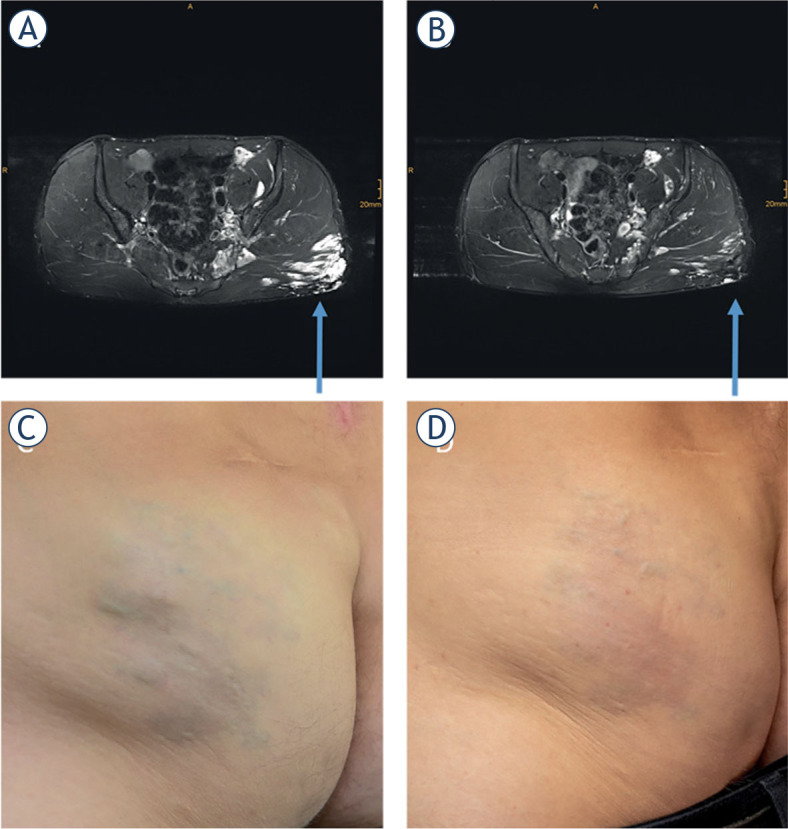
Patient treated by BEST. Axial T2-weighted, fat-saturated MRI with hyperintense (arrow) gluteal venous malformation before treatment (A). Axial T2-weighted, fat-saturated MRI of the same region 4 months after treatment. The main part of the venous malformation is occluded (B). Photography before (C) and after the treatment (D).
